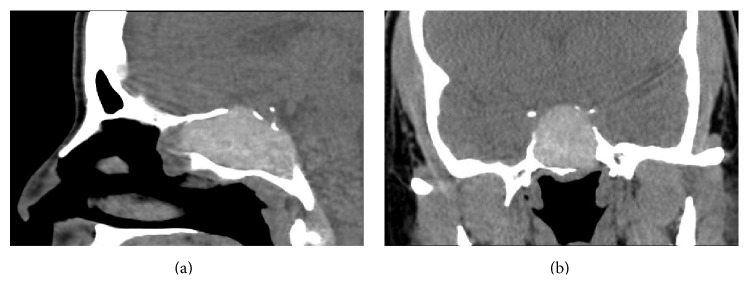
Sinus CT with sagittal (a) and coronal (b) reconstructions demonstrating a 5.0 × 2.7 × 2.5 cm hyperdense sphenoid mass with marked sphenoid expansion, suggestive of allergic fungal sinusitis. There is erosion of the sella, clivus, and a portion of the planum sphenoidale.
